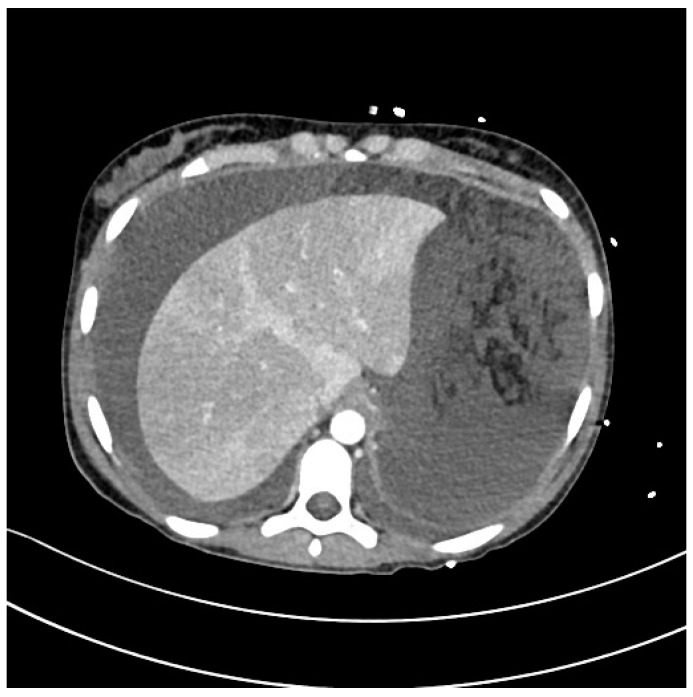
CT scan of bilateral pleural and ascitic effusions and impaired liver density.
